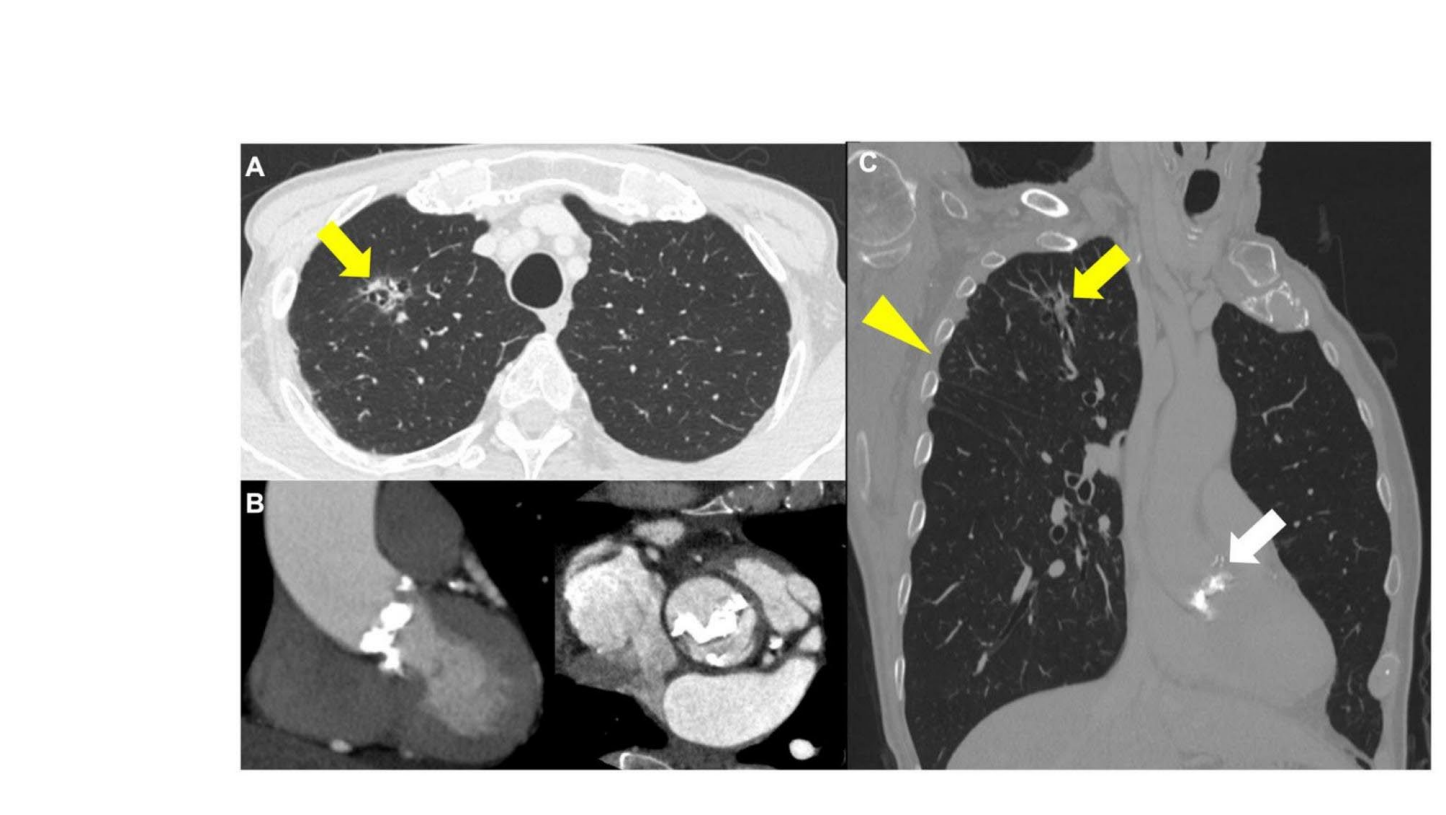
Preoperative CT findings. A: a nodular shadow in the right upper lobe without enlarged lymph nodes or distant metastases (yellow arrow). B: a severely calcified type 0 bicuspid aortic valve stenosis. C: the relationship lung cancer (yellow arrow), aortic valve(white arrow) and 4th intercostal space (yellow arrow head)
